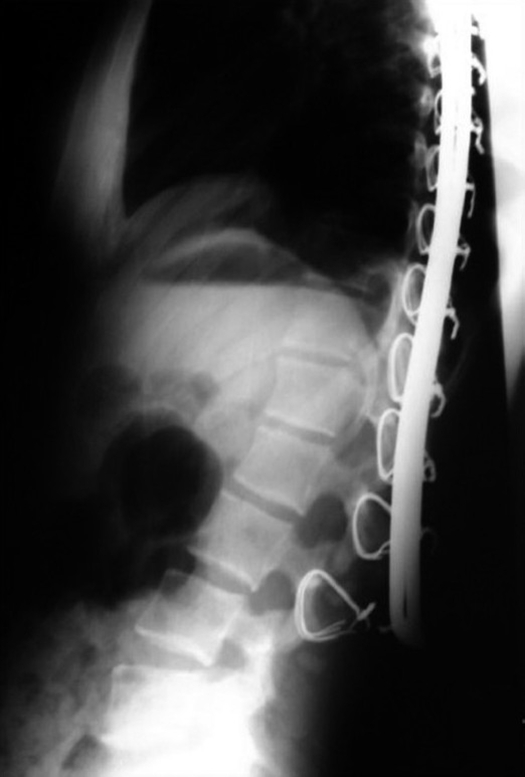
Lateral X-ray of dorsolumbar spine shows wire cut outs due to undercontouring of Hartshill rectangle
